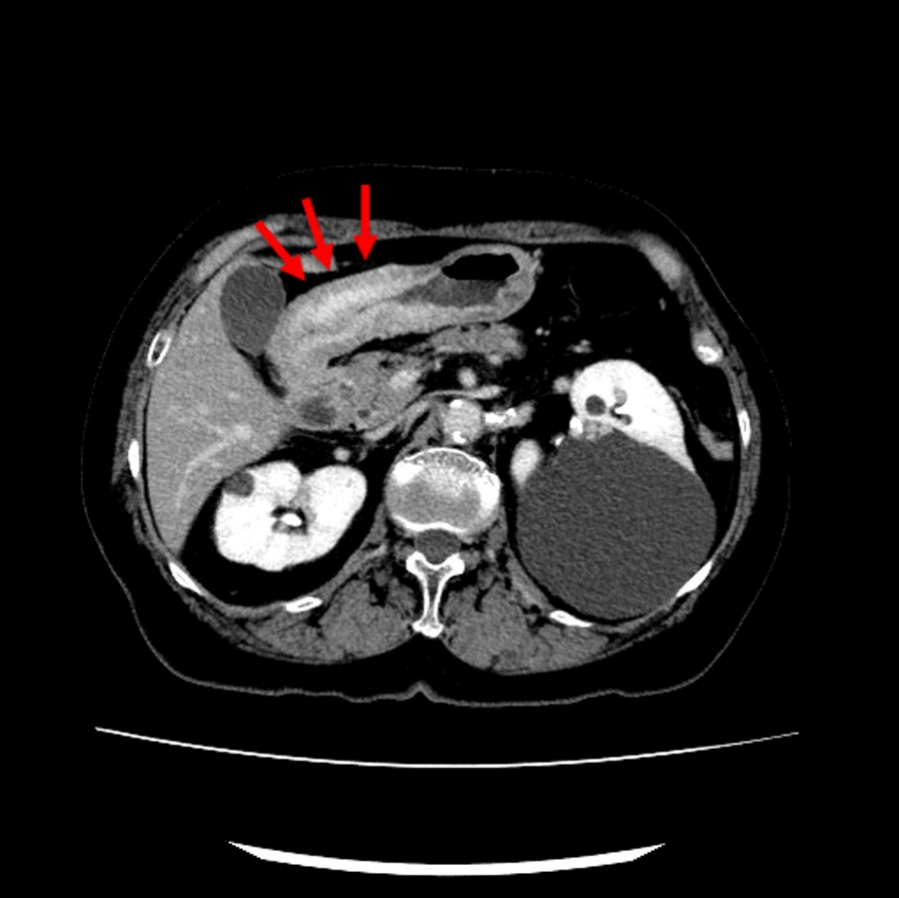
Computed tomography revealed enhanced wall thickening in the lesser curvature of the stomach from the antrum to the middle of the body (red arrows)
